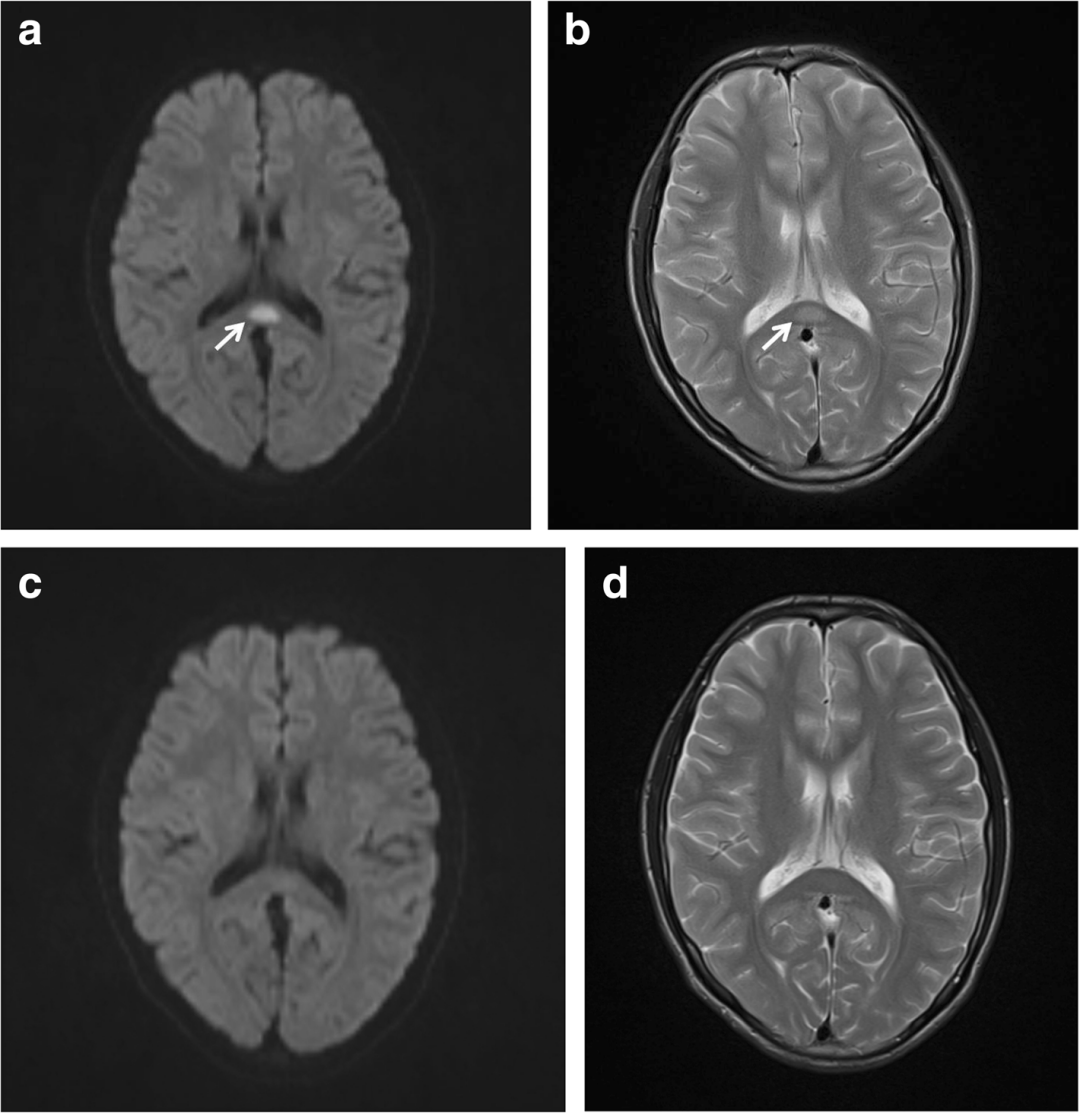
The brain magnetic resonance imaging (MRI) in case 1. The brain MRI on day 3 after admission revealed high intensity lesions (arrows) in the splenium of the collupus callosum (SCC) on diffusion- (a) and T2- weighted images (b), which disappeared on day 7 (c and d)
